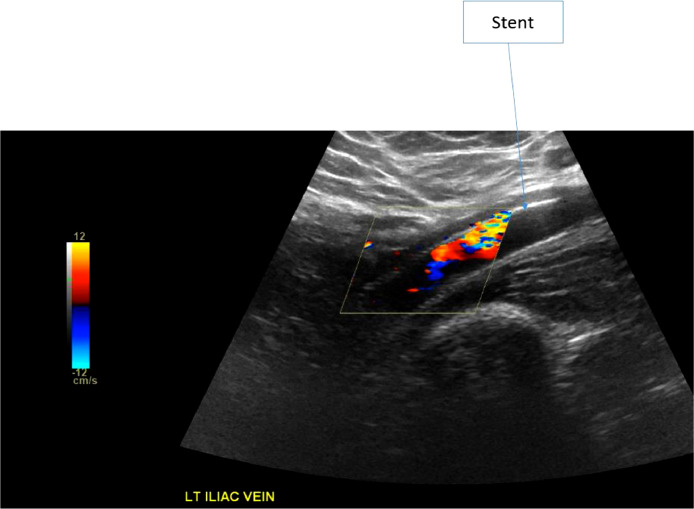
Venous Doppler Ultrasound of the left iliac vein with self-expanding stents.
